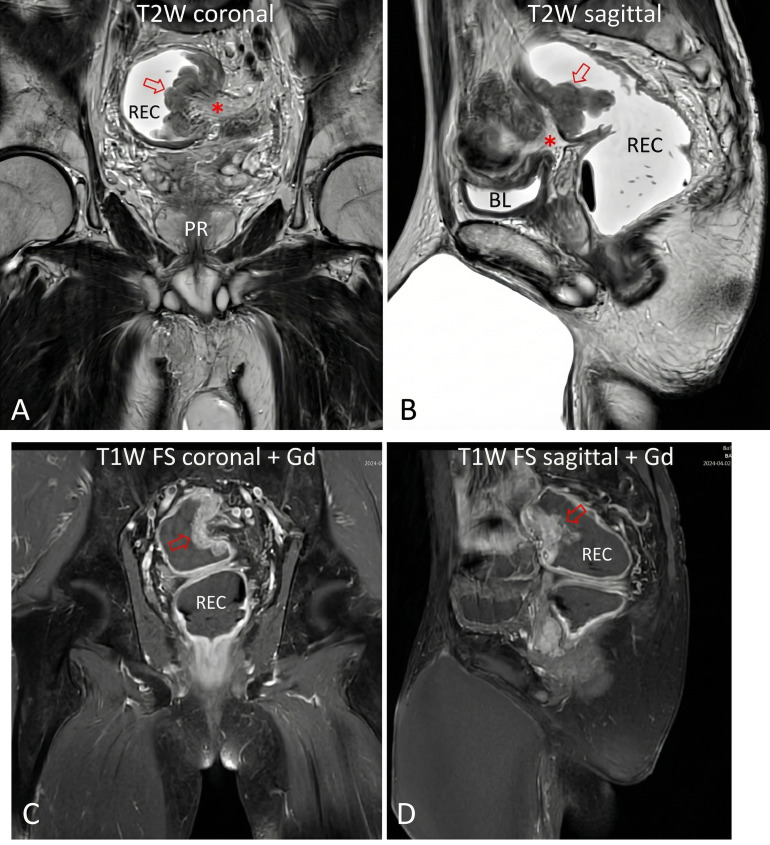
Pelvic MRI with various pulse sequences and planes: (A, B) T2W coronal and sagittal images demonstrate abnormal thickening of the left anterior wall of the middle third of the rectum over a 6-cm segment, forming an intraluminal mass with an irregular, lobulated surface and hyperintensity relative to the bladder muscle. (C, D) Post-gadolinium contrast images show strong, heterogeneous enhancement of the lesion with mild infiltration of the perirectal fat layer. REC, rectum; PR, prostate; BL, bladder; T1W, T1-weighted; T2W, T2-weighted; T1W FS, T1-weighted fat-saturated; Gd, gadolinium.
